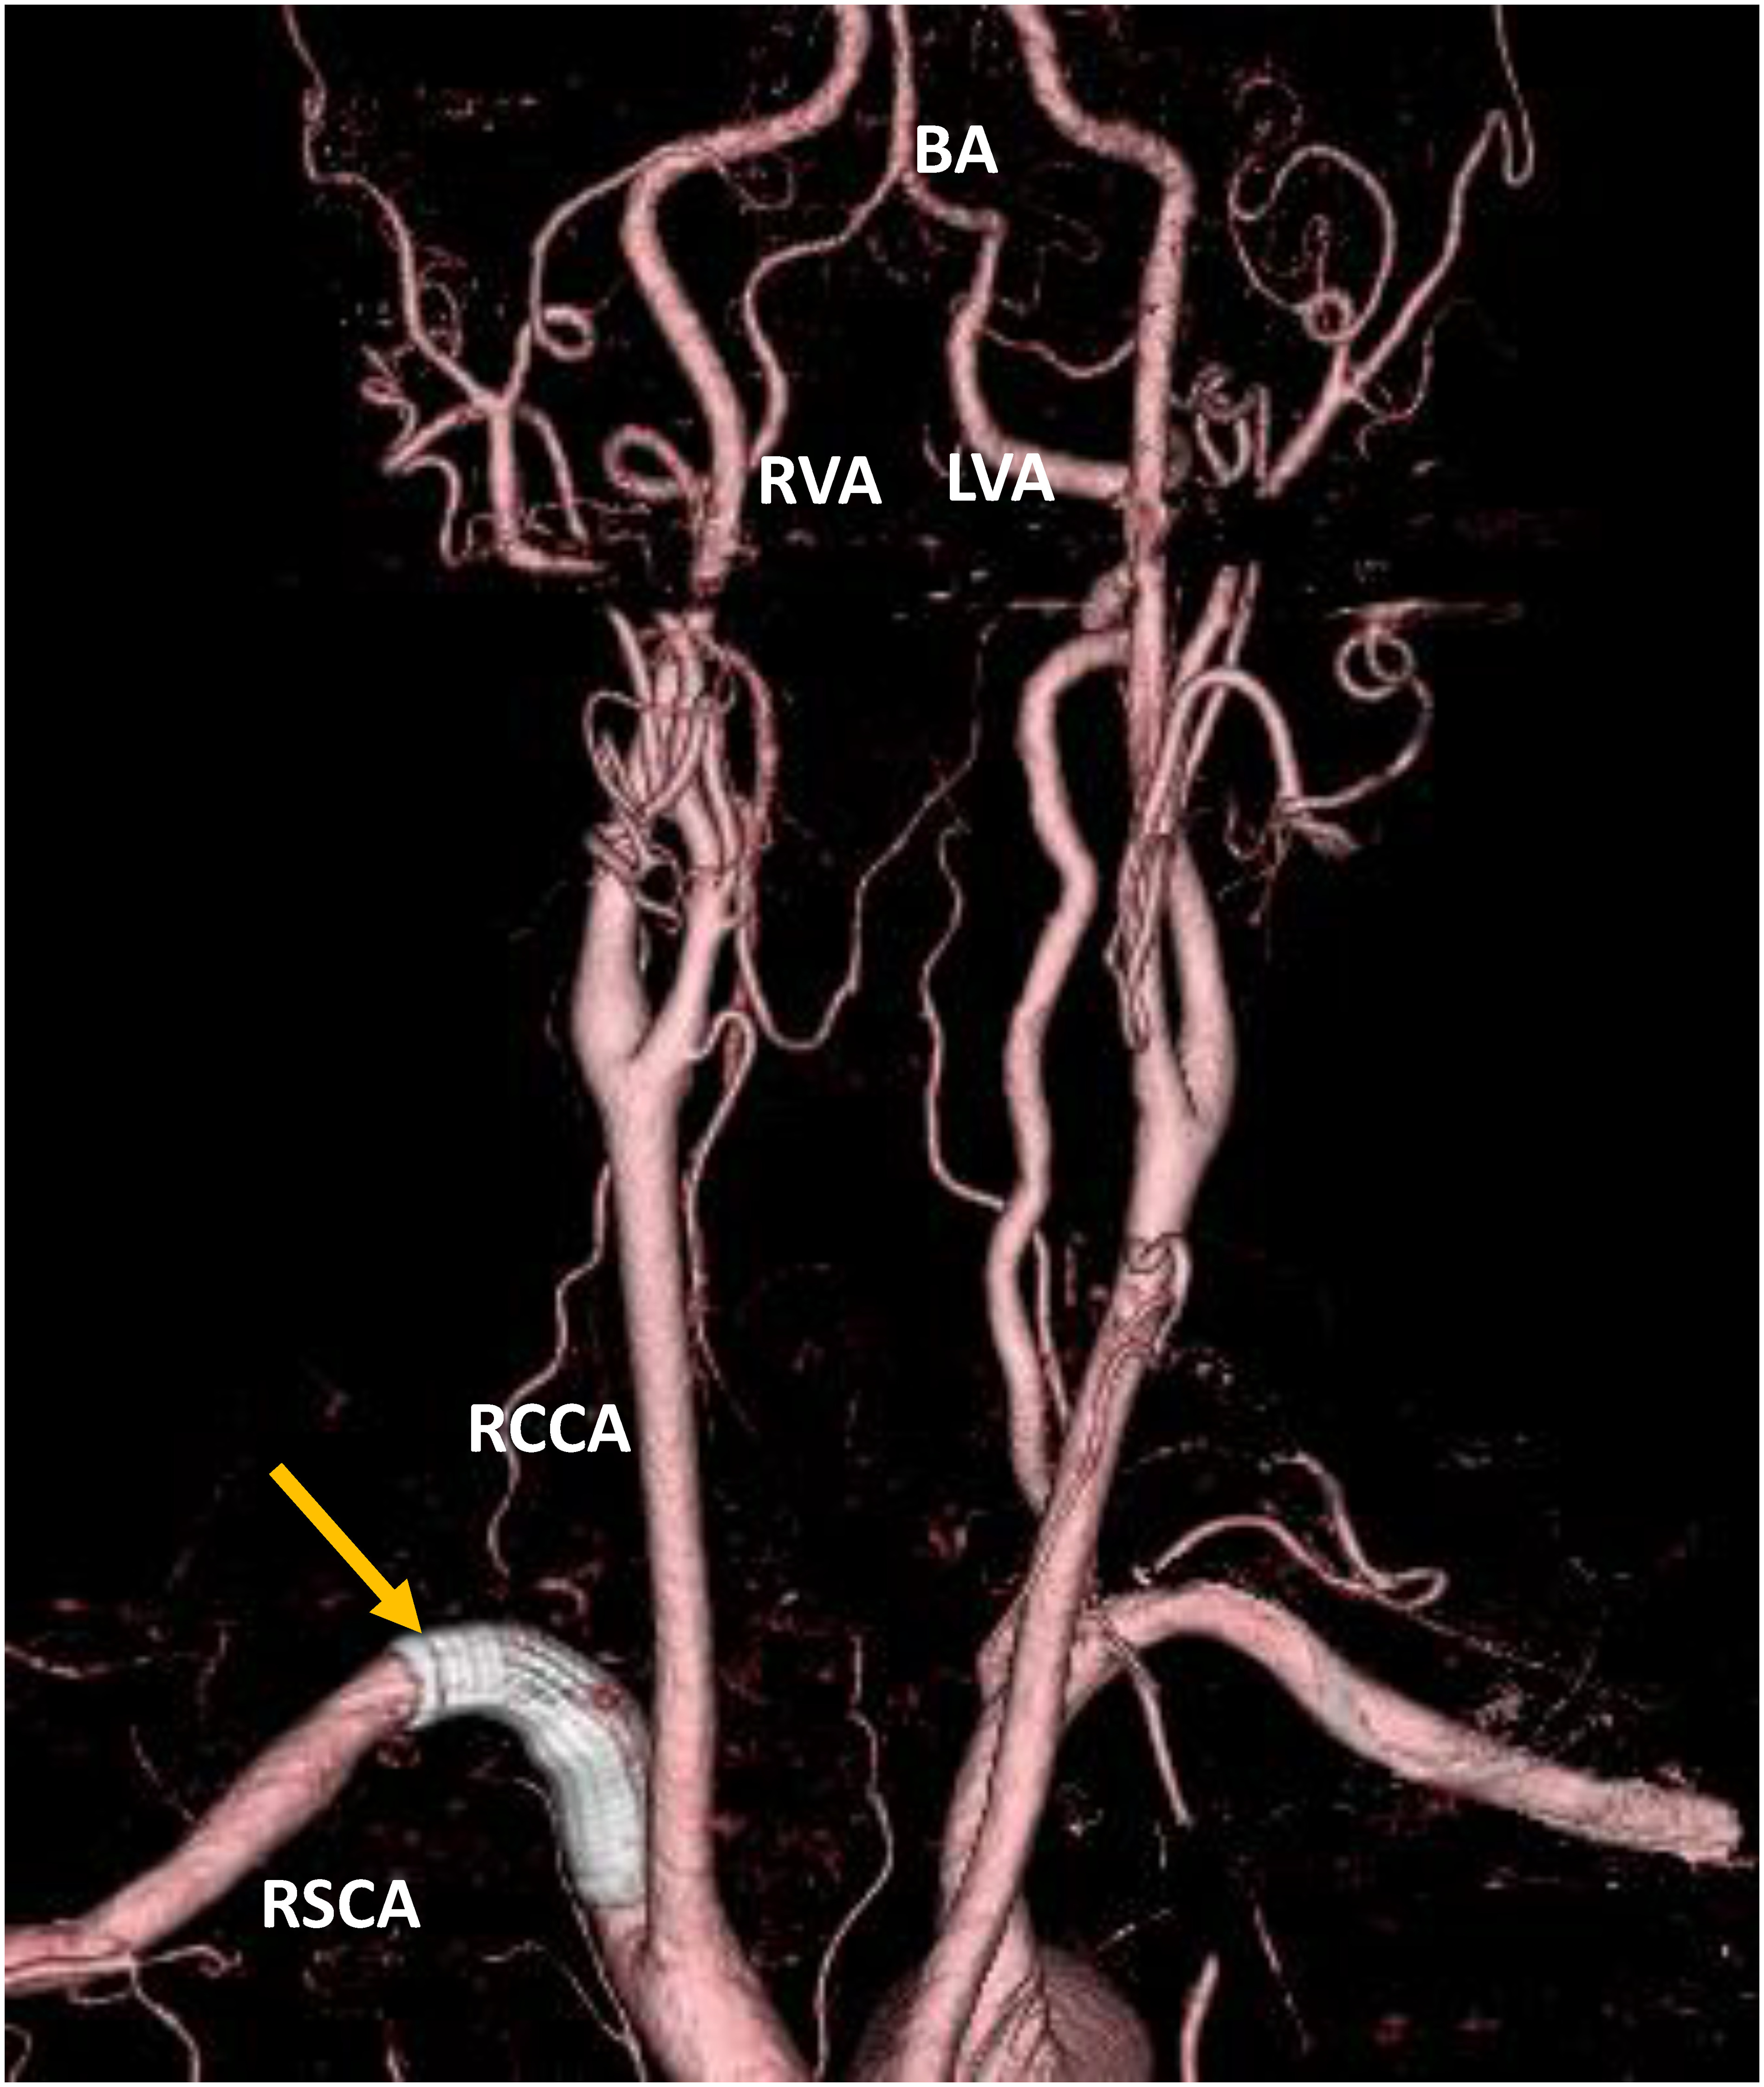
Fig. 3 Postoperative computed tomography. The arrow presented the covered stent.
